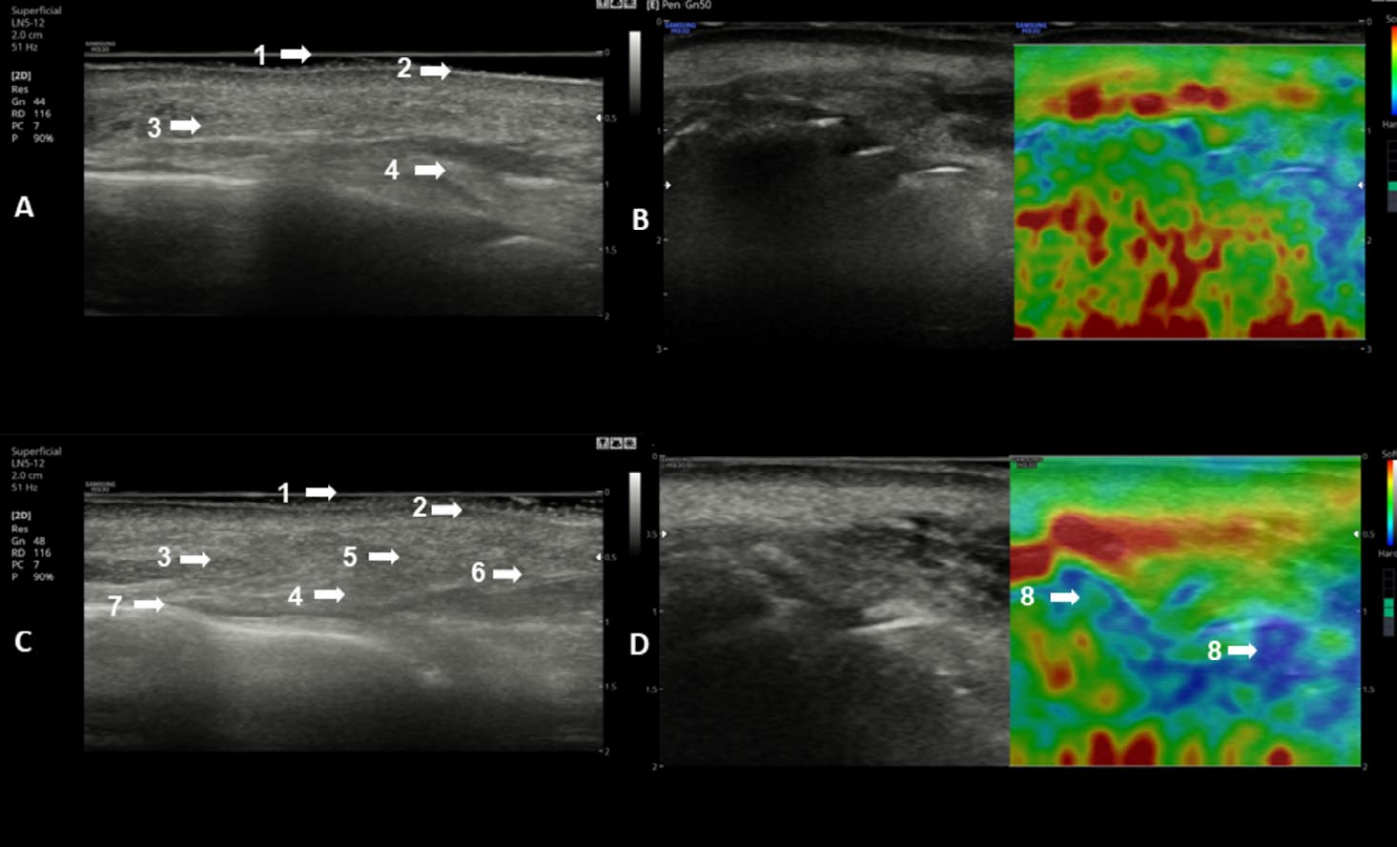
Ultrasound images of the right (R) nasolabial folds at baseline (A) and at 6 months post‐initial treatment (C). Qualitative elastography images of the same region are shown at baseline (B) and at 6 months (D) in the patient presented in Figure 4. At baseline (A), ultrasound revealed a heterogeneous pattern of the nasolabial fold. By month 6 (C), there was a notable increase in tissue density at NLF points 1–2–3, evidenced by the following changes: A reduction in the Sub‐Epidermal Low Echogenic Band (SLEB), accompanied by an increase in echogenicity, indicating higher tissue density (5, 6), despite the persistence of some heterogeneity (7). Evidence of neocollagenesis at the level of the reticular dermis, particularly at its interface with the subcutaneous tissue (5, 6). Areas of increased viscoelasticity, corresponding to a higher elastic modulus, were visualized in blue on qualitative elastography (8).
